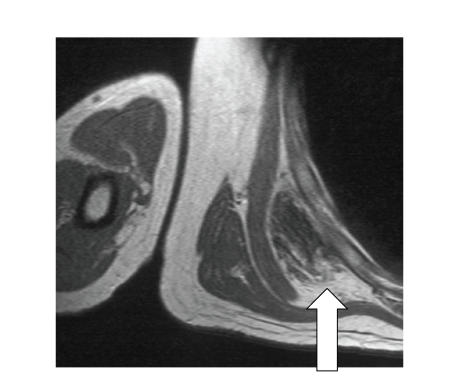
Axial T1-weighted MR image showing an elastofibroma as a
